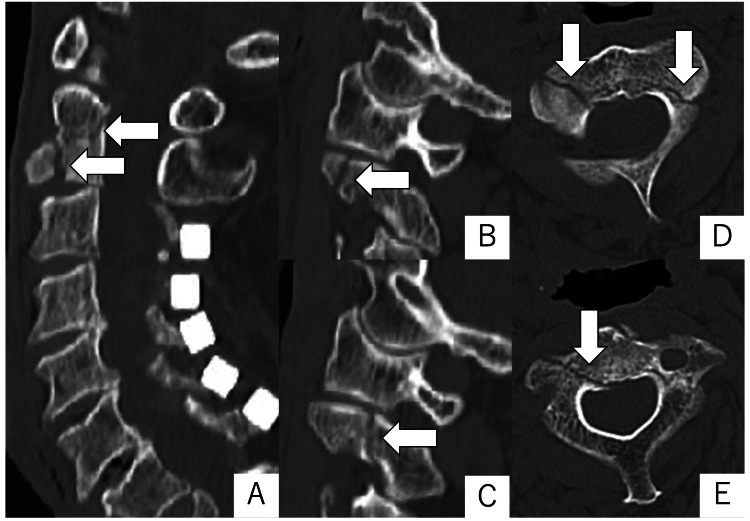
CT images two weeks after the injury. CT imaging two weeks after the injury revealed an enlarged fracture line and dislocation in progression (arrows). A-C: sagittal; A: midline; B: right; C: left; D, E: axial.
